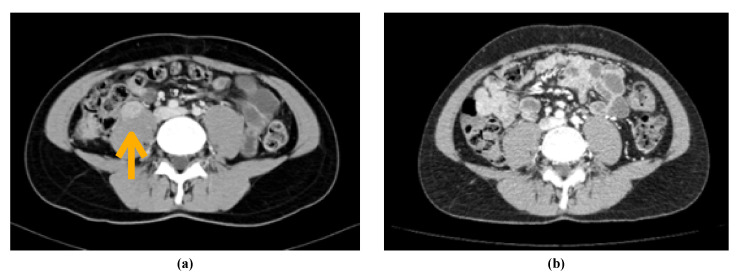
CT scans showing the evolution of the oncological disease. (a): CT confirming second progression. Increase of retroperitoneal node and a new node anterior to the right psoas. (b): CT with major partial response. Shows stable retroperitoneal node and resolution of the rest of metastatic disease.
